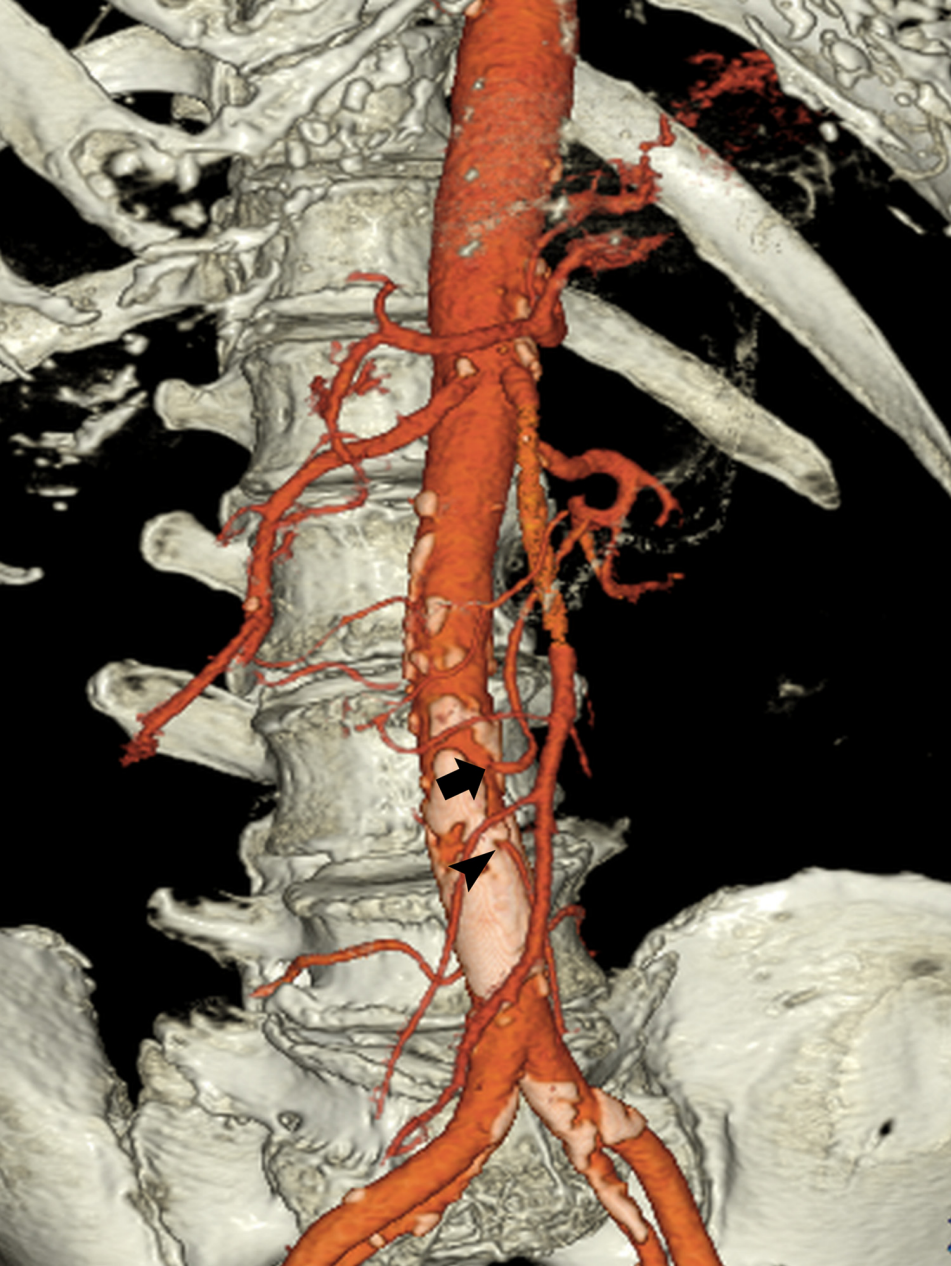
3D-computed tomography. The middle mesenteric artery (arrow) originates from 12 mm cranial to IMA (arrow head). IMA, inferior mesenteric artery.
